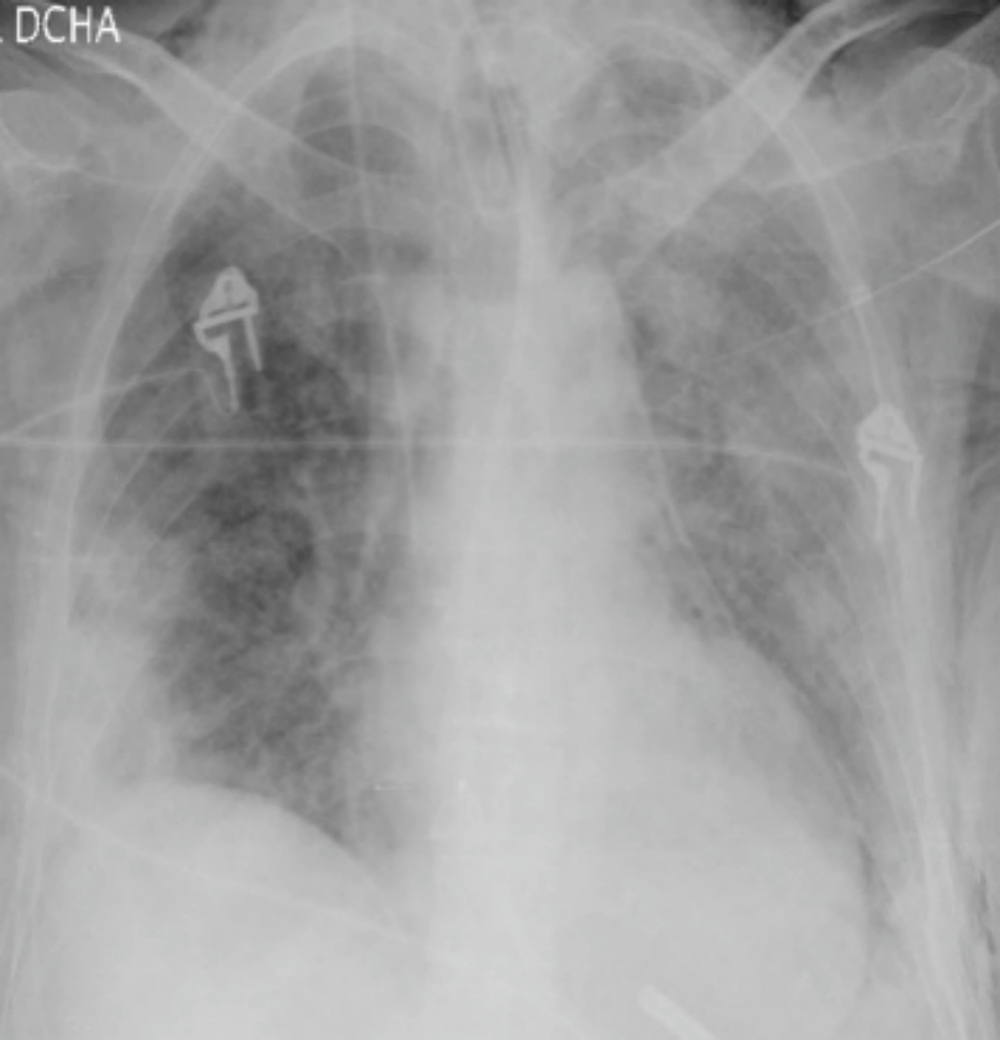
Chest x-ray at hospital admission Chest x-ray showing bilateral pulmonary infiltrates
